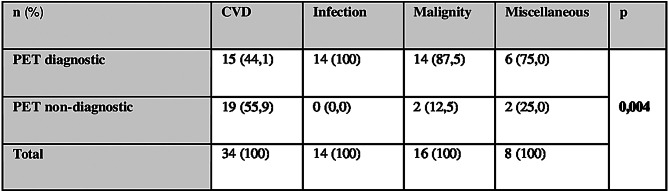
Contribution of PET-CT results to diagnosis according to FUO etiologies
